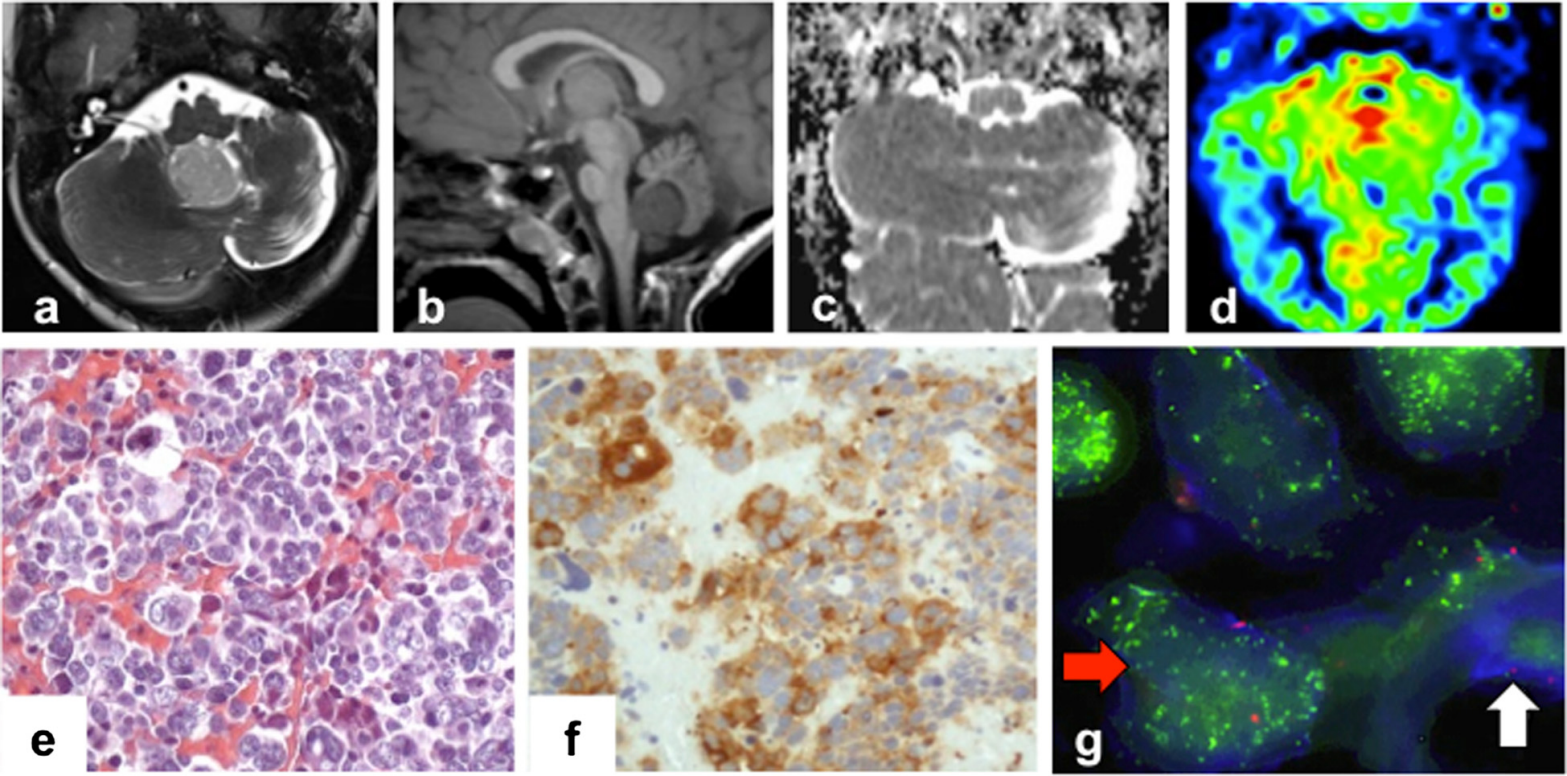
Imaging and Histopatological features of MB2. MRI. Axial T2-weighted image (a) and sagittal T1-weighted image (b) show a nodular mass originating from the vermis and bulging into the fourth ventricle. Apparent diffusion coefficient (ADC) map (c) shows lack of diffusion restriction. Perfusion weighted imaging (d) reveals hyperperfusion within the lesion. (e) Tumor is characterized by pleomorphic cells with large nuclei, prominent nucleoli and moderate eosinophilic cytoplasm. (f) Large cells show immunoreactivity for synaptophysin. (g) N-Myc oncogene amplification (green spots) detected in neoplastic nuclei (blue) and centromere 8 signals (red spots) using CEP8/BAC as FISH probes. Red arrow: N-Myc amplified cell. White arrow: N-Myc dyploid cell
